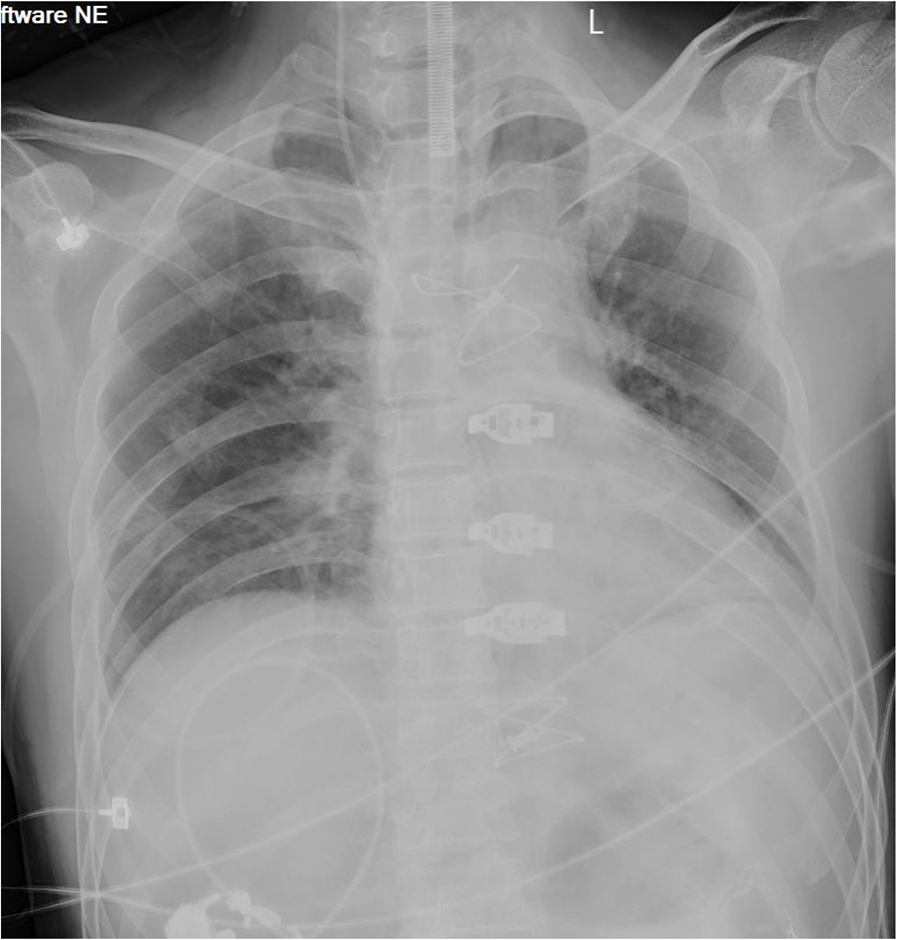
Postoperative radiograph revealed no residual high-density shadows
